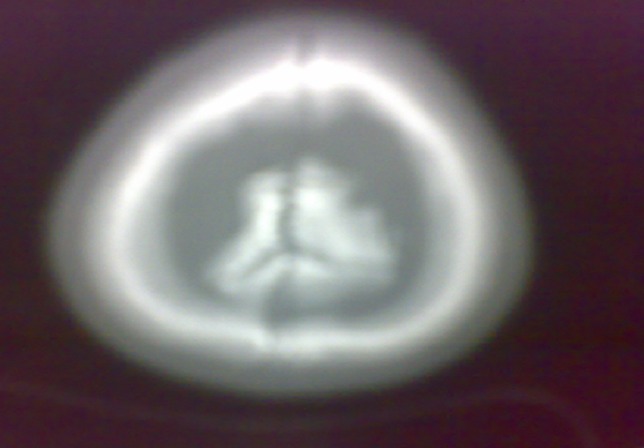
Bone window of coronal CT scan showing depressed fractures of bilateral parietal bones in high parietal region
